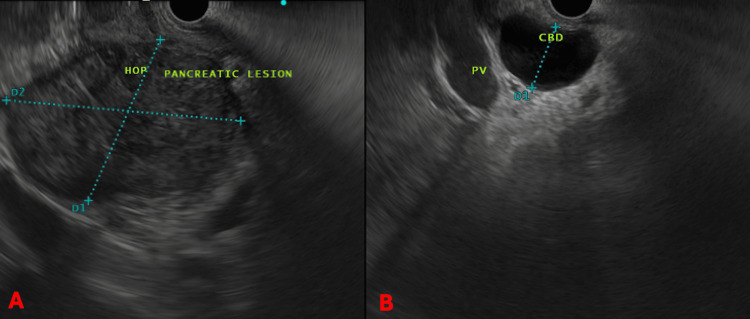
(A) Endoscopic ultrasound (EUS) showing a large mass in the pancreatic head. (B) EUS showing a dilated common bile duct. HOP: head of pancreas; CBD: common bile duct; PV: portal vein.
